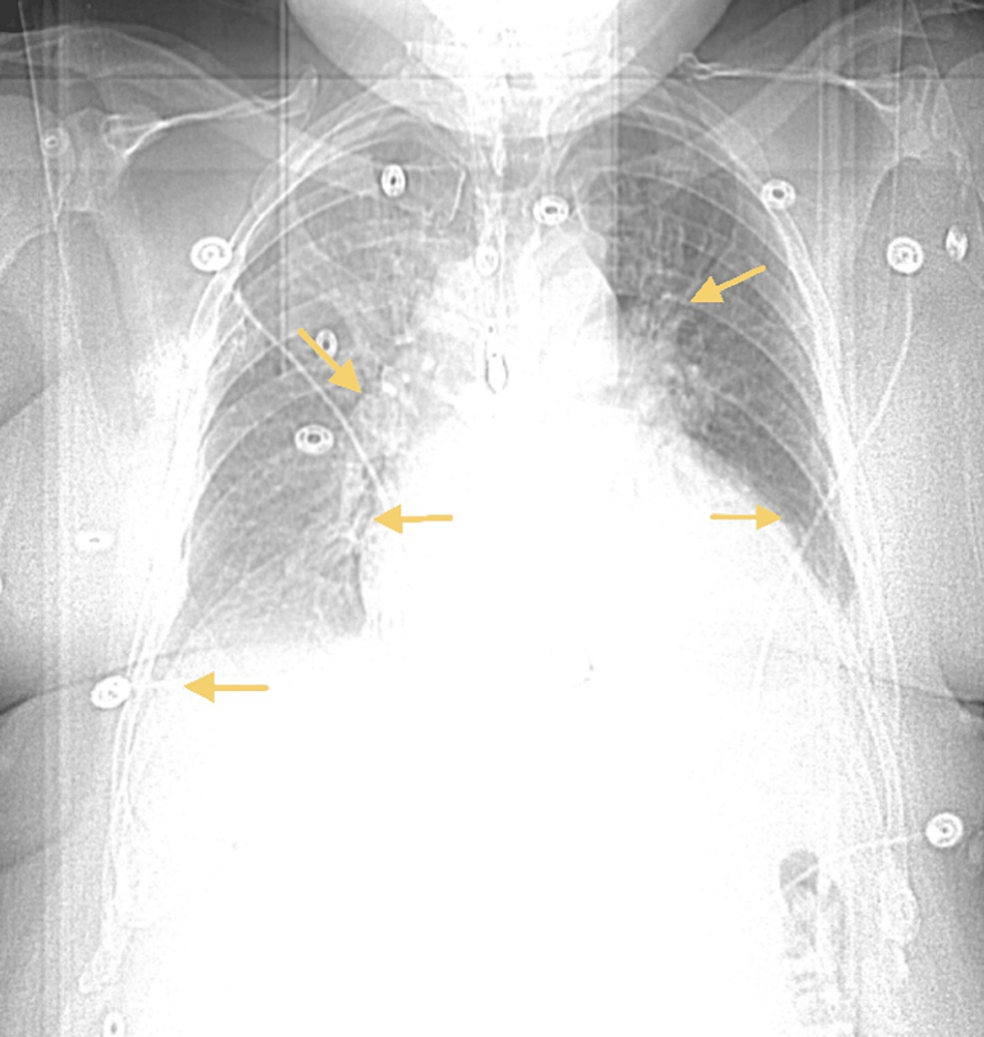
Chest radiograph coronal view. (a) Moderate volume bilateral pleural effusions with overlying atelectasis (superior arrows). (b) Rounding and enlargement of myocardium, evidencing inflammation, and accumulation of pericardial fluid (medial arrows). (c) Blunting of costophrenic angles (inferior arrow).
